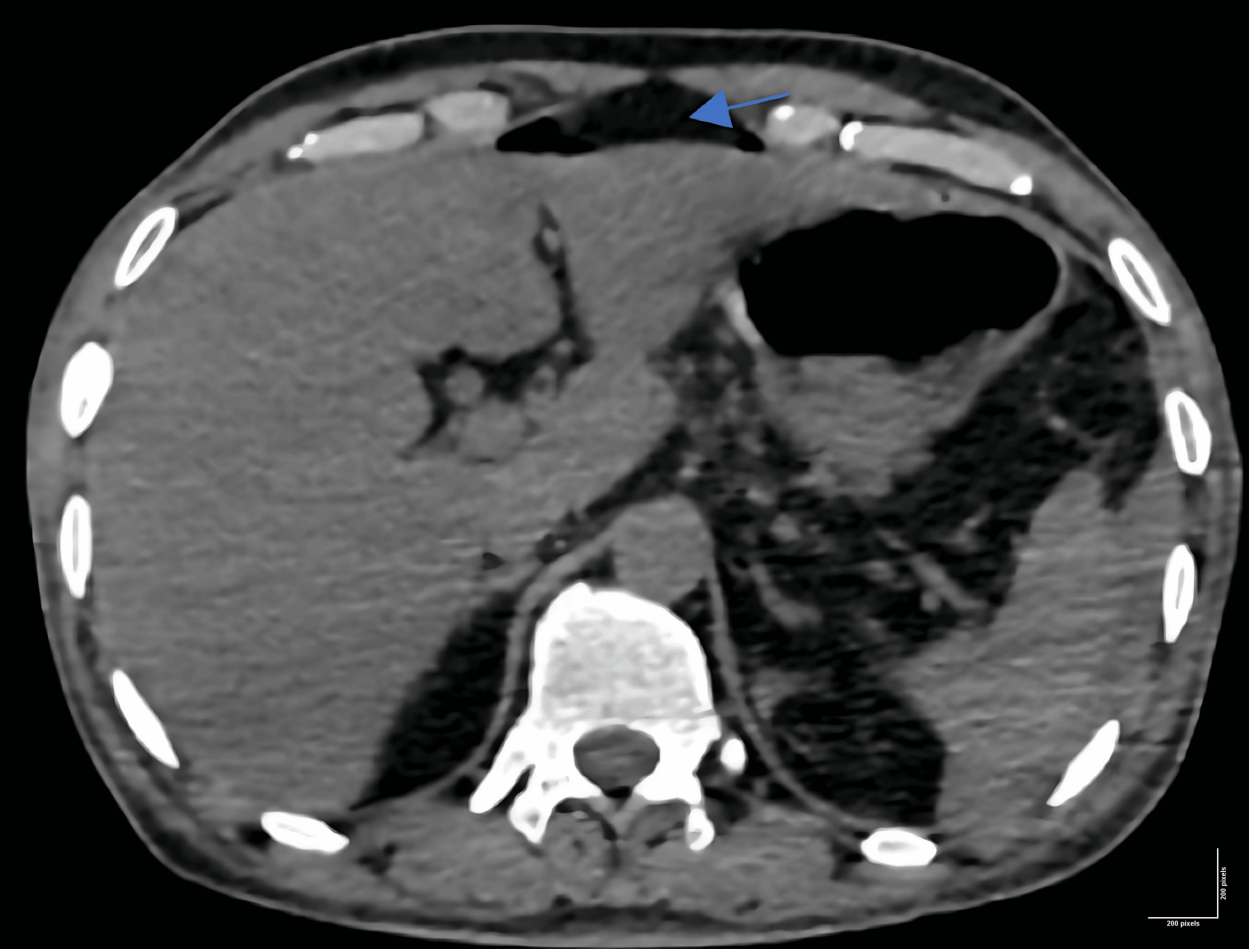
CT scan shows pneumoperitoneum (arrow) with an absent gall bladder.
